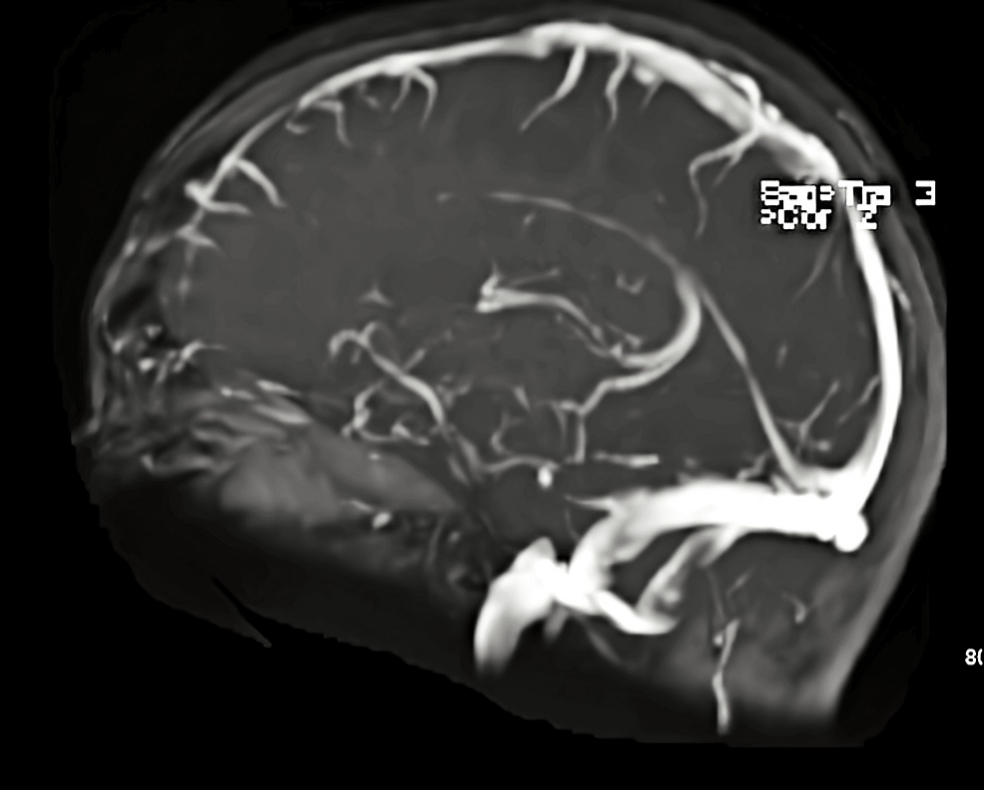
MRI venogram negative for sinus thrombosis.
